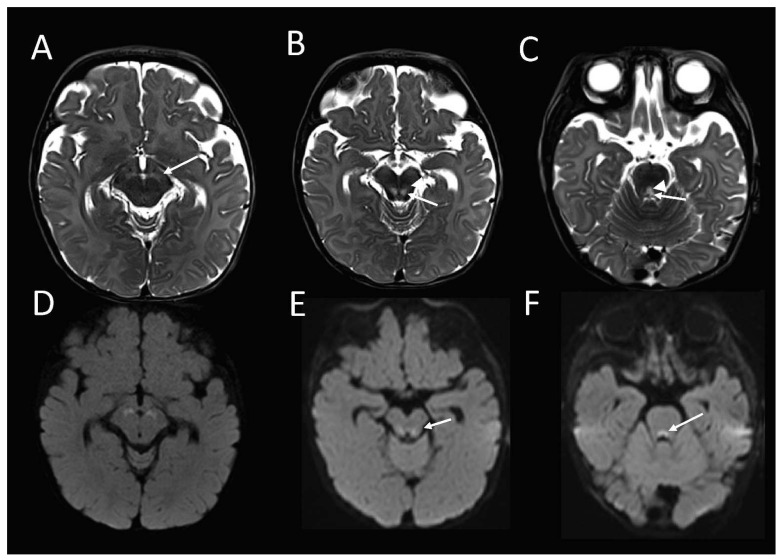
Brain MRI from the patient with compound heterozygous *PYROXD2* variants at 4 ½ months. The axial T2-weighted sequence shows abnormally high signal in the (A) substantia nigra; (B) midbrain tegmentum, involving the central tegmental tracts (arrow), and the medial lemnisci (arrowhead) and; (C) upper dorsal pontine tegmentum, involving the tectospinal tracts (arrowhead) and middle longitudinal fasciculi (arrow), with corresponding restricted diffusion (D–F) on diffusion-weighted imaging.
